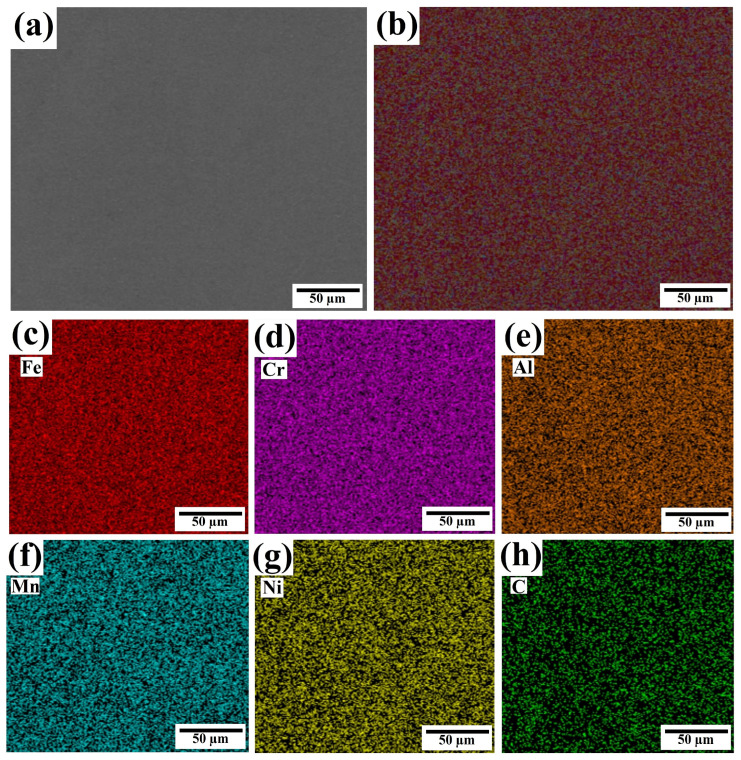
EDS mapping of the R-UVALC coating at 5 µm ultrasonic amplitude, (a) selected portion of the R-UVALC coating surface, (b) element distribution mapping of the selected portion of the coating, and (c–h) element distribution mapping of each element Fe, Cr, Al, Mn, Ni, and C, respectively.
